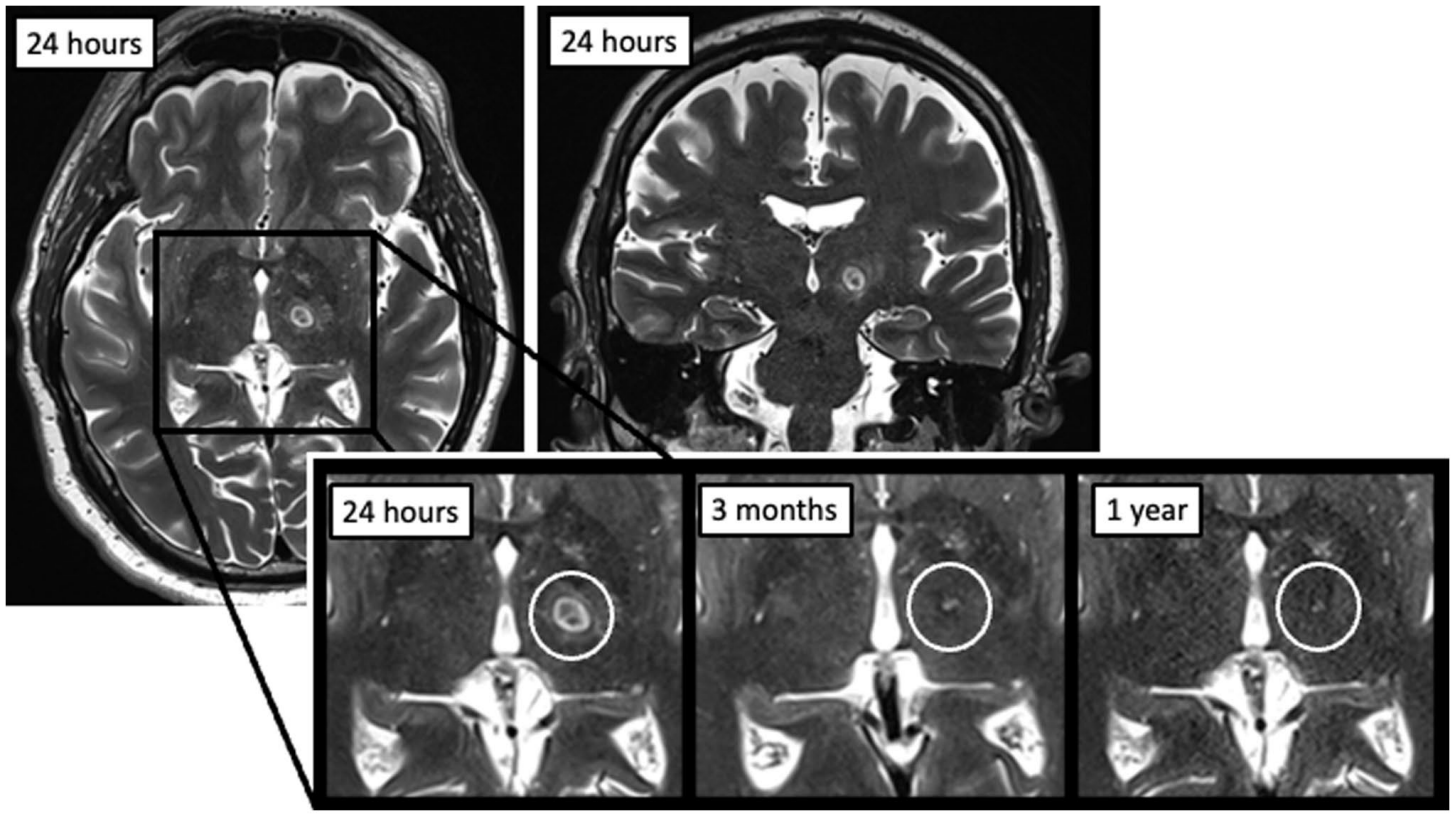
Example of an MRgFUS thalamotomy lesion at 24 h post-procedure on axial (left) and coronal (right) T2-weighted imaging, as well as evolution over time at three time points (24 h, 3 months, and 1 year).
